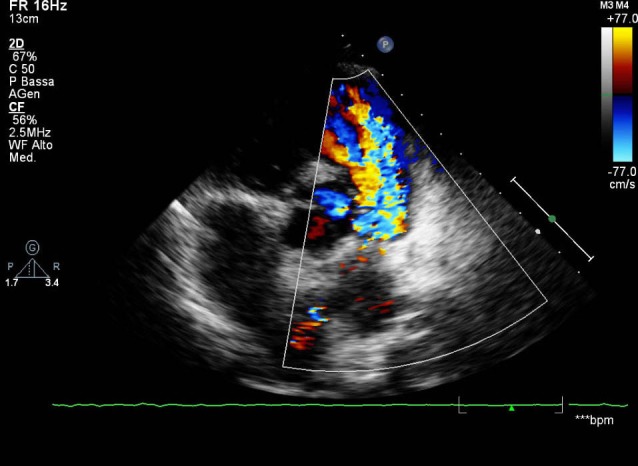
The transthoracic echocardiogram revealed an aneurysmatic left sinus of Valsalva, with a remarkable turbulent flow moving from the proximal aortic root to the right outflow tract.
